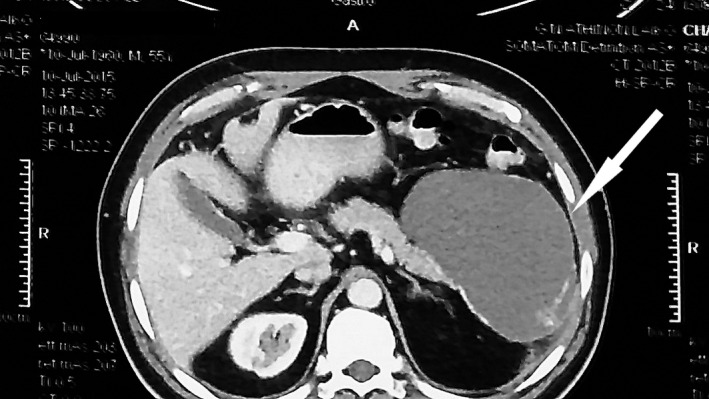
Computed tomography revealing a cystic mass at the tail of the pancreas
